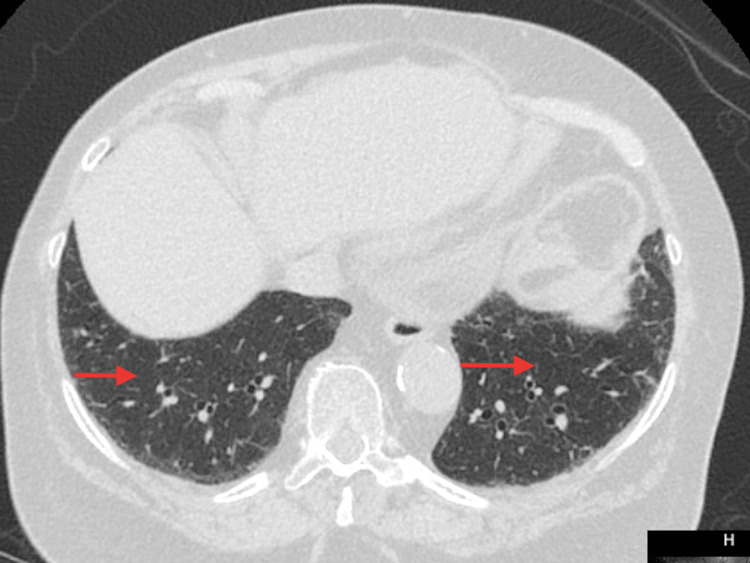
High-resolution CT chest at follow up clinic visit showing near complete resolution of pleural-based lesions and basal ground glass opacities
